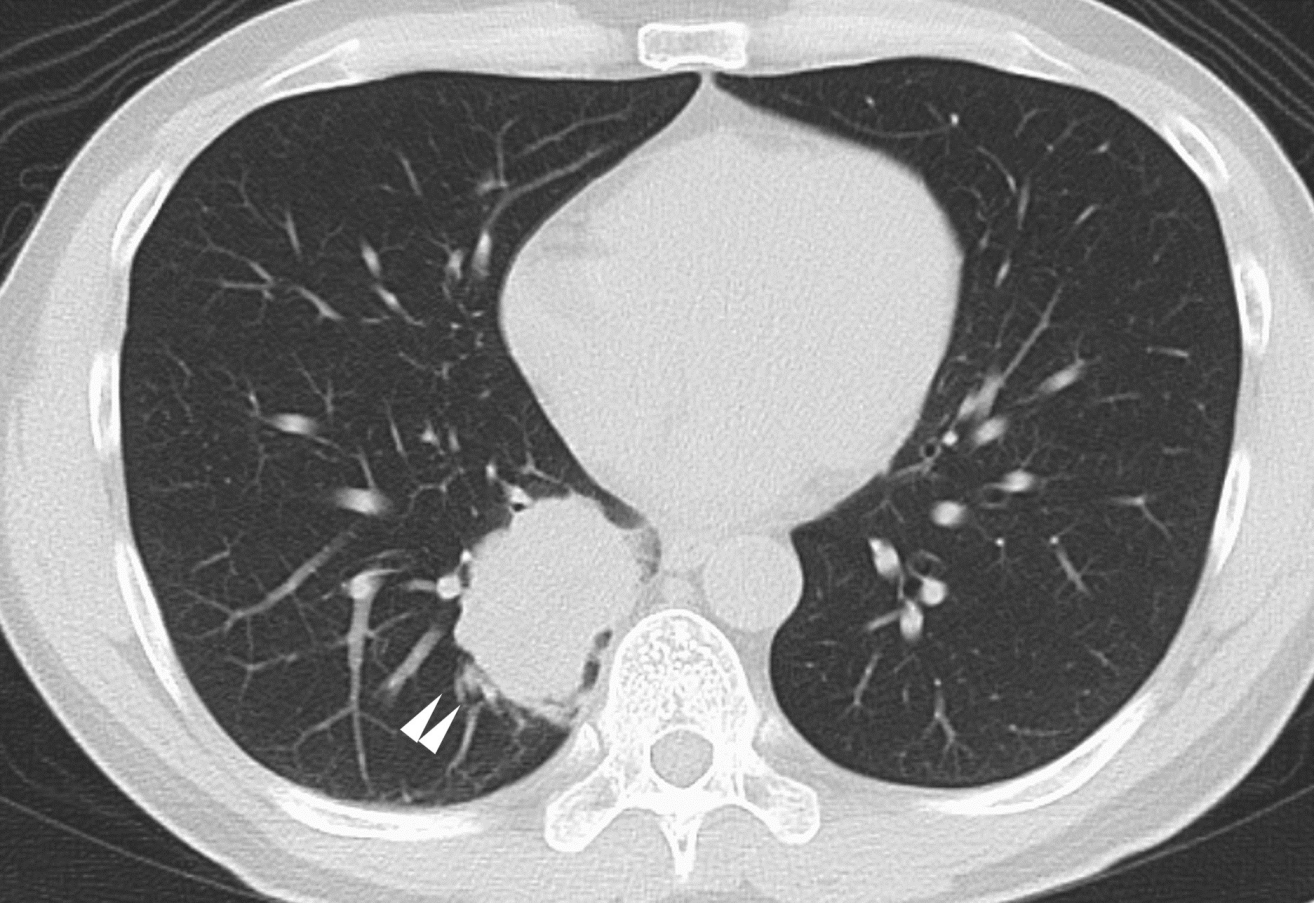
Chest computed tomography for the diagnosis of lung cancer. The white arrow points to a 53 × 39 mm lesion.
